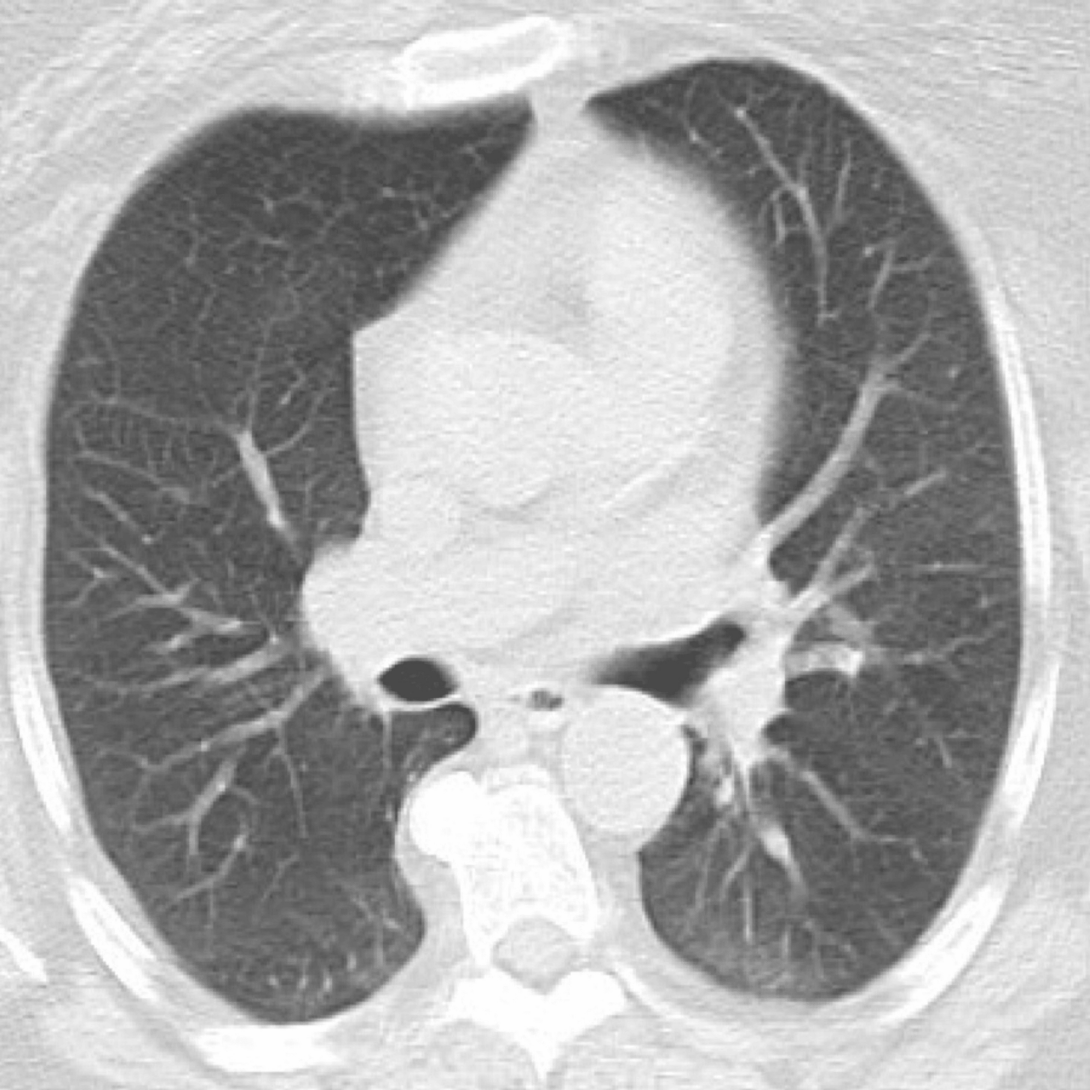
CT scan of the chest without contrast
